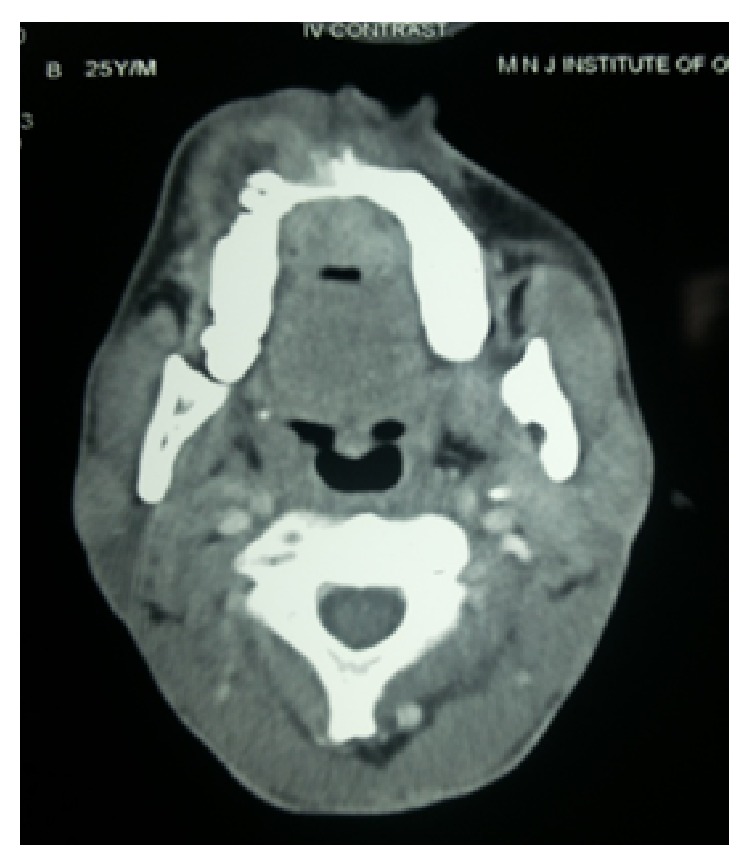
Axial CT scan showing ill-defined enhancing soft tissue mass on anterior hard palate extending posteriorly up to the soft palate on right side.
